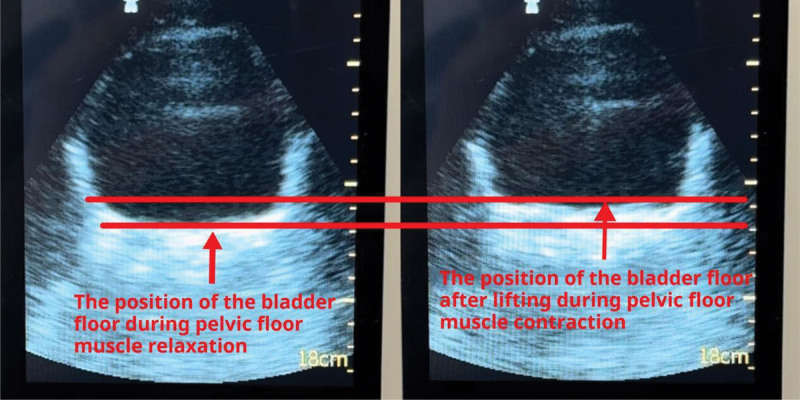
Observation of bladder floor elevation during pelvic floor muscle contraction using ultrasound.
